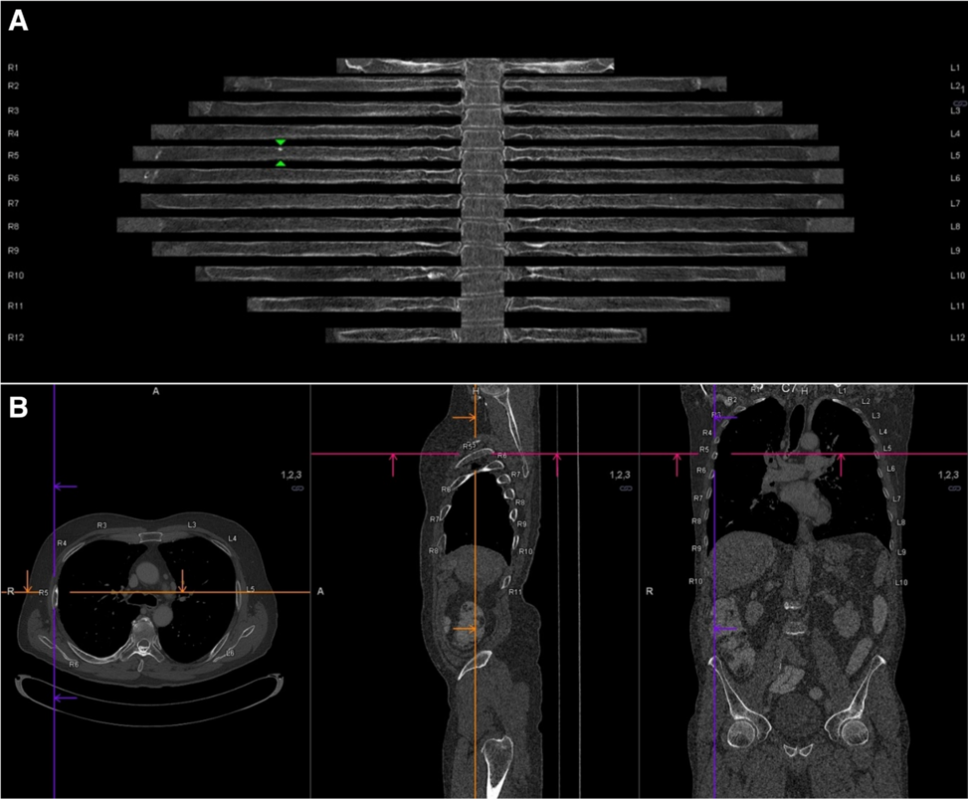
a Example of a post-processed in-plane image of the ribs. A small sclerotic lesion is visible in the 5^th^ right rib (*green arrows*). b Multiplanar display of the corresponding thoraco-abdomino-pelvic CT-scan (*bone window*) in axial, sagittal and coronal planes, with a small sclerotic lesion in the 5^th^ right rib (localized with hairline crosses)
